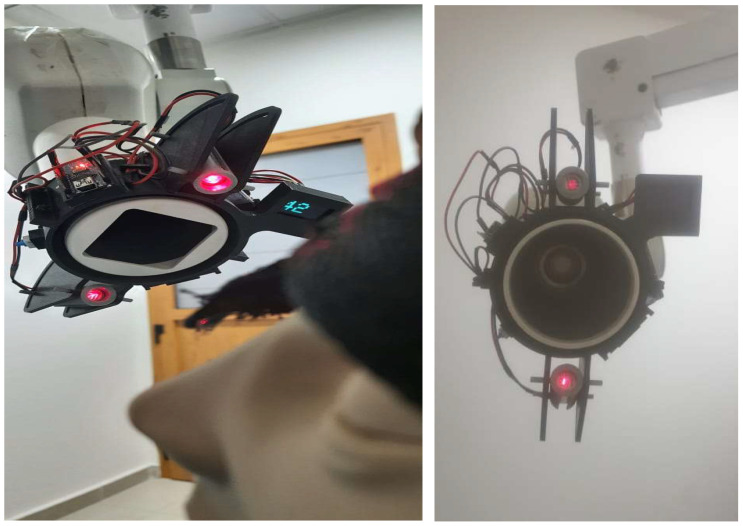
Nallan’s Direct Ray gyroscopic-guided intraoral radiographic device shown in two views during positioning. The red dots represent active status LEDs used for alignment and stabilization feedback. The green element corresponds to the digital display indicating device operating parameters.
